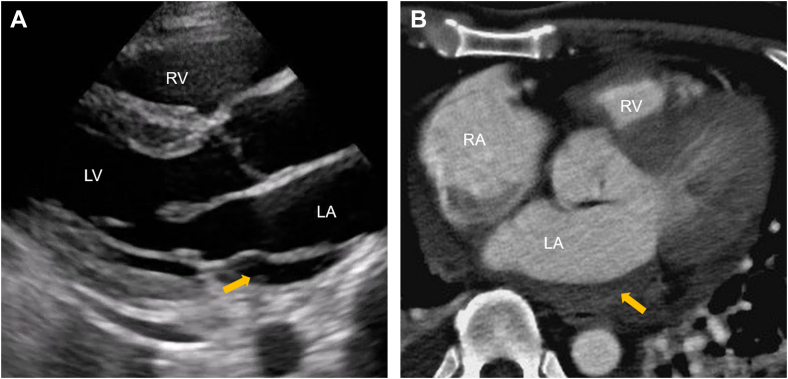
Transthoracic Echocardiography and Cardiac CT on Postoperative Day 4 (A and B) TTE and cardiac CT showed a sudden decrease in the size of the LAIH (arrow). TTE = transthoracic echocardiography; other abbreviations as in Figure 4.
